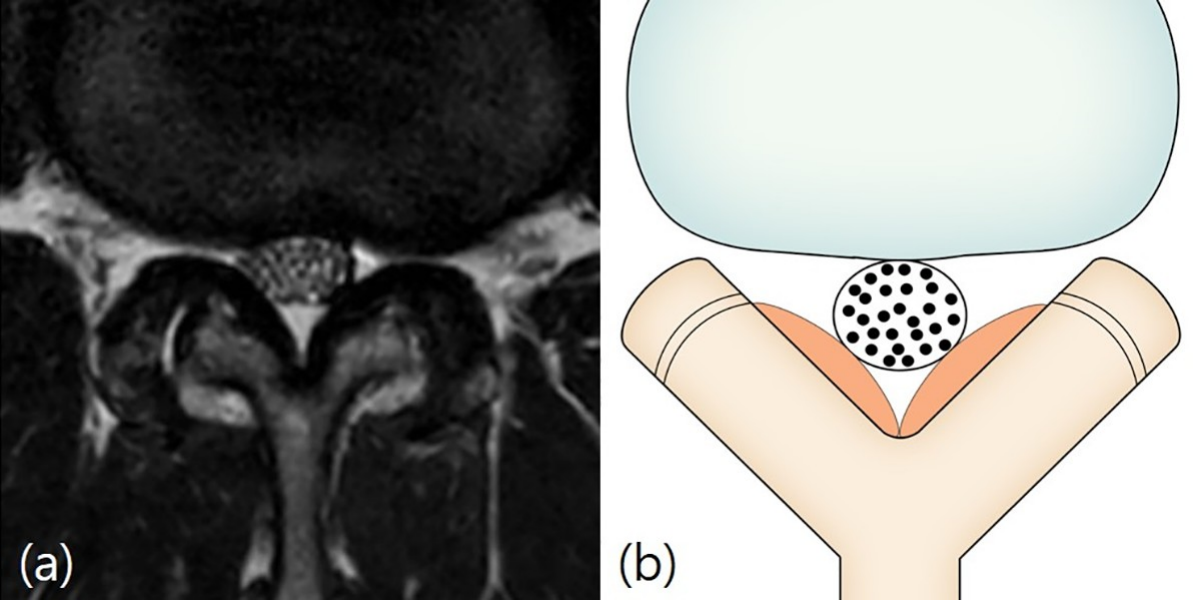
Schizas classification. Grade B (moderate stenosis). Fig 2A and 2B: A 59-year-old man with pain in both buttocks and both lower extremities. T2-weighted axial magnetic resonance image at L2/3 disc level shows the rootlets occupying the entire dural sac, but rootlets can still be individualized.
